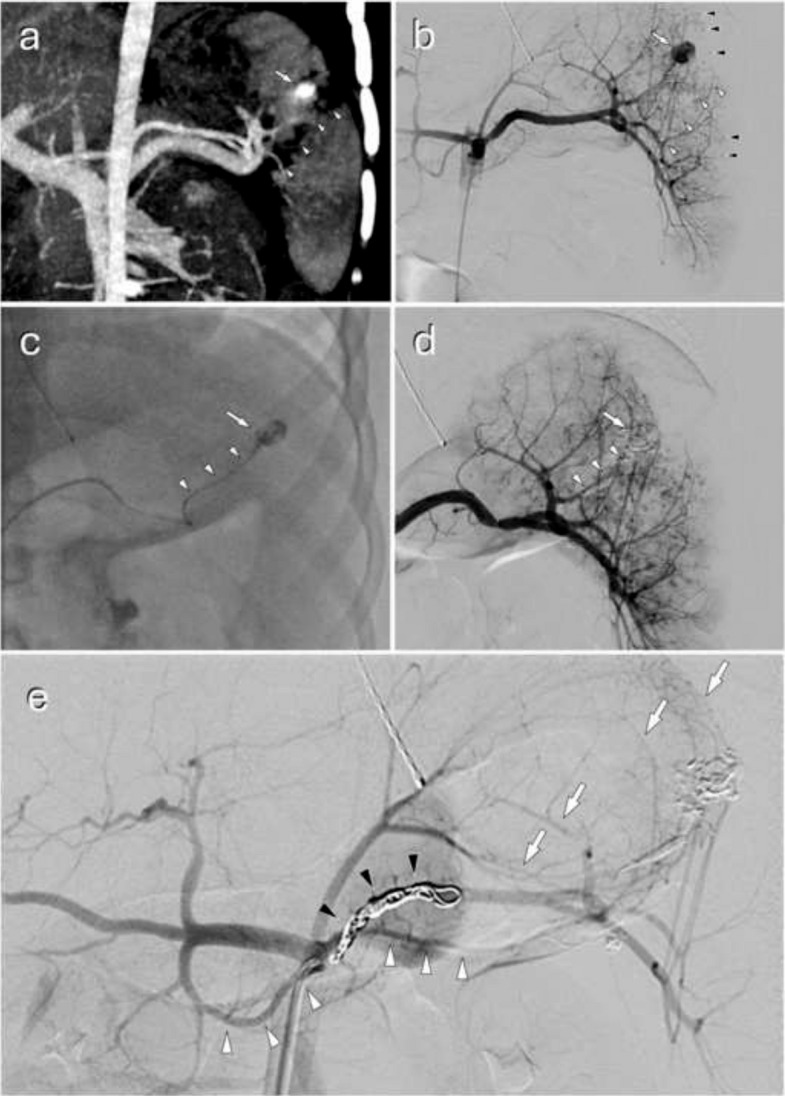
A spleen trauma managed by distal selective and truncal embolization. A 6-year-old patient following a bicycle fall with abdominal trauma. a Contrast-enhanced CT revealed a grade IV splenic injury according to the *American Association for the Surgery of Trauma (AAST)* classification, characterized by a 4 cm through-and-through laceration extending to the hilum (arrowheads), associated with hemoperitoneum and an 8 mm splenic pseudoaneurysm (arrow). The patient was hemodynamically stable. After multidisciplinary discussion, a decision was made to proceed with interventional radiology management. b Under general anesthesia, a coeliac angiography confirmed CT findings, with 8 mm pseudoaneurysm (arrow), laceration extending to the hilum (white arrowheads), and demonstrated diffuse splenic parenchymal petechiae (black arrowheads). c During and d after superselective microcatheterization and embolization of both the pseudoaneurysm (arrow) and its feeding branch (arrowheads) using Onyx-18 (Medtronic, Irvine, CA, USA). e Truncal embolization was then performed using 7 mm and 4 mm Concerto coils (Medtronic, Irvine, CA, USA) (black arrowheads). Post-embolization angiography demonstrated reperfusion of the splenic parenchyma via collateral flow through the dorsal pancreatic artery (white arrowheads), as well as short gastric vessels (arrows)
